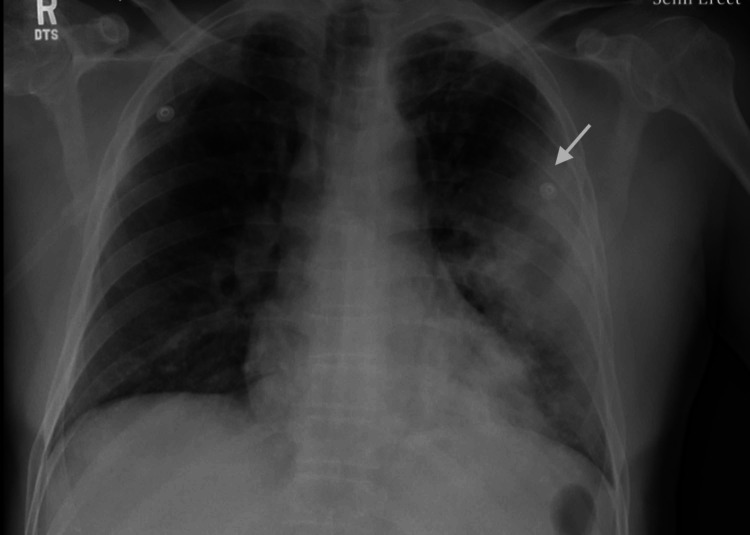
Initial CXR of the patient The image shows large airspace consolidation in the left mid-lung with a cavitary appearance (arrow), and bi-apical reticular opacities, more prominent on the left than right CXR: chest X-ray
